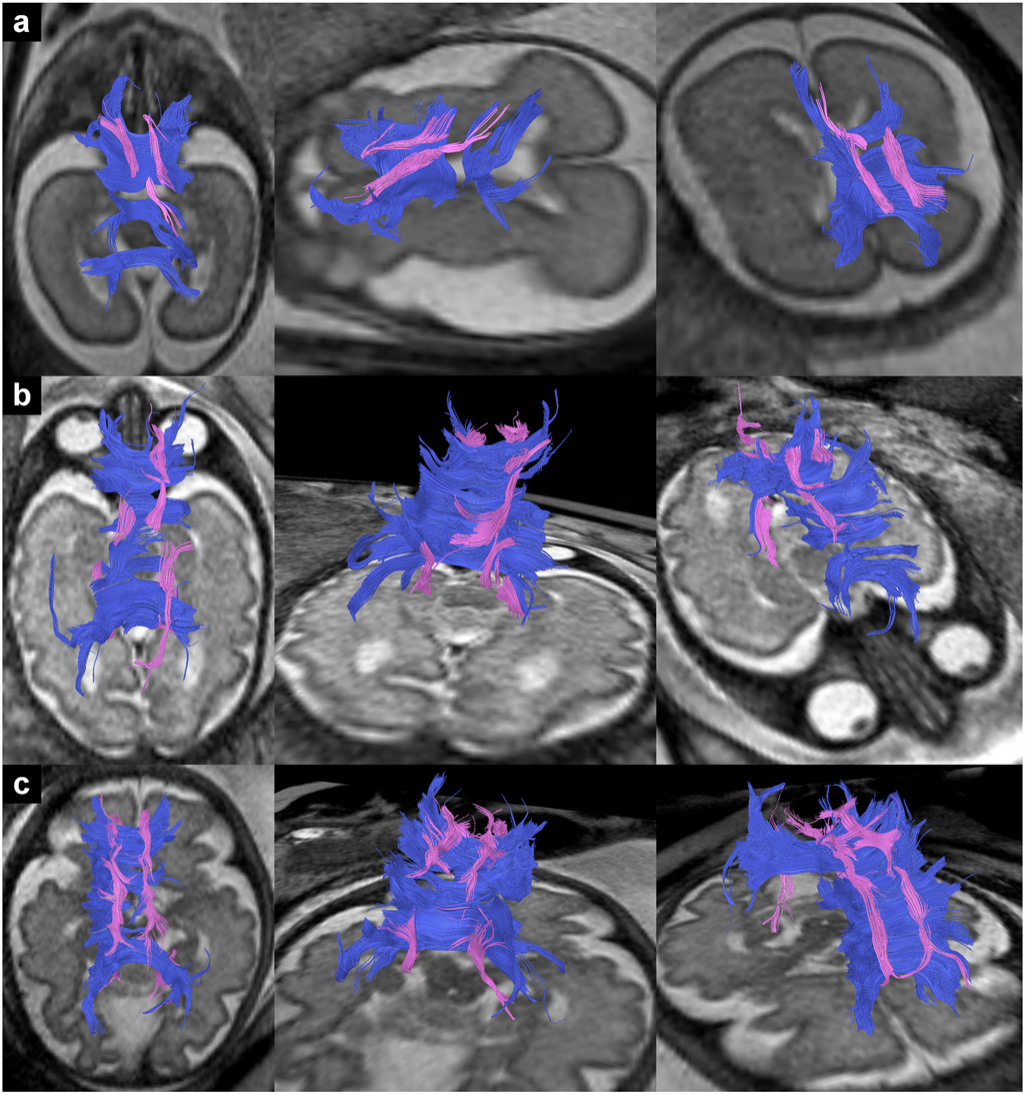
In utero tractography of the cingulum (pink) in subjects 15 (a), 20 (b) and 23 (c) (27, 33 and 33 GW respectively) and its relation to the corpus callosum (dark blue). Tractography of the corpus callosum was performed according to the methods described in Kasprian et al. (2008) [26]. Fiber tracts are projected on axial T2-weighted images.
